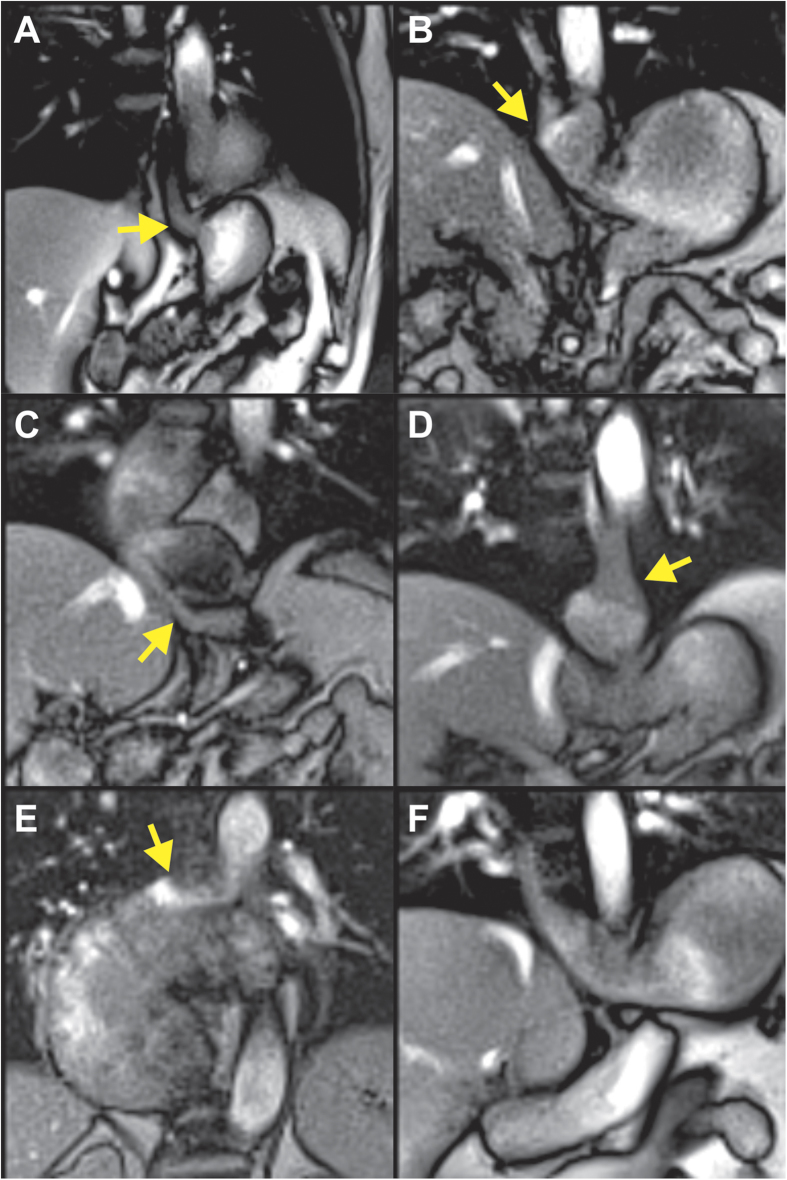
Real-time MRI of gastroesophageal swallowing in patients with (A) reflux, (B) hiatal hernia, (C) achalasia, (D) telescoping oesophagus after fundoplication, (E) thoracic stomach, and (F) functional heartburn without reflux evidence. Individual images (50 ms acquisition time, 2 × 2 mm^2^ resolution, 8 mm section thickness) were selected from corresponding movies (S11 to S17 in Supplementary Appendix). Arrows indicate (A,B) bolus regurgitation from the stomach into the oesophagus during Valsalva maneuver and (C–F) bolus transport from oesophagus into the stomach (C–F).
